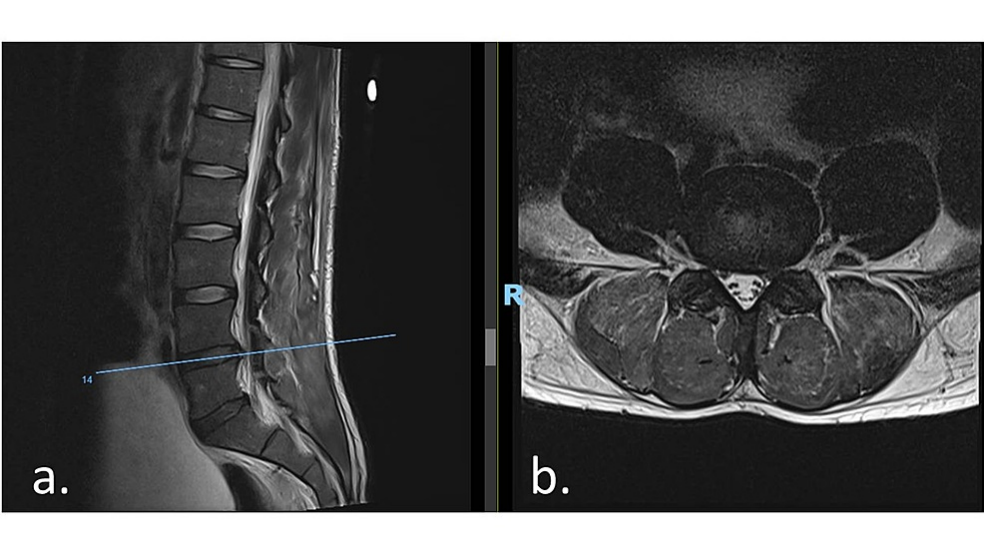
MRI lumbar spine showing an L4/5 diffuse disc bulge, with mild bilateral subarticular zone stenosis with possible mild compression of the left descending L5 nerve root A distended urinary bladder is noted. (a) Sagittal cut. (b) Axial cut. MRI: magnetic resonance imaging, R: right.
